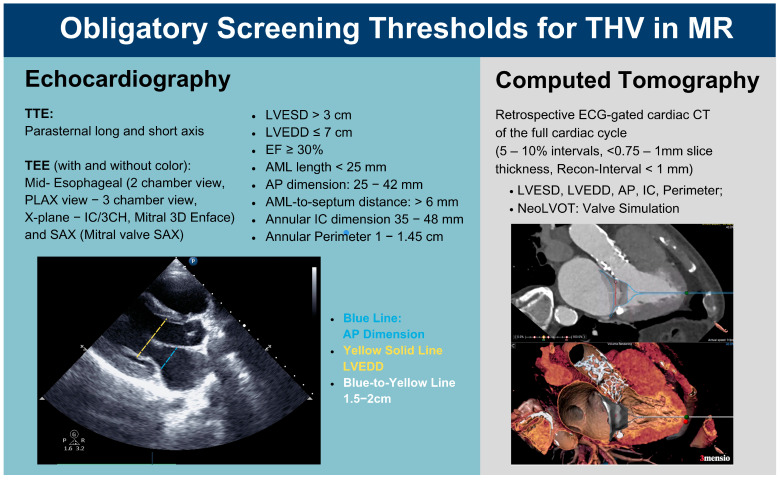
Obligatory screening thresholds for THV on MR. AML, Anterior Mitral Leaflet; AP, Anterior–Posterior; CT, Computed Tomography; EF, Ejection Fraction; IC, Intercommissural; IC/3CH, Intercommissural 3 Chamber View; LVEDD, Left Ventricular End-Diastolic Diameter; LVESD, Left Ventricular End-Systolic Dimension; MR, Mitral Regurgitation; NeoLVOT, Neo Left Ventricular Outflow Tract; PLAX, Parasternal Long-Axis; SAX, Short-Axis; TTE, Transthoracic Echocardiography; TEE, Transesophageal Echocardiography; THV, Transcatheter Heart Valve (Abbott Prescreening Guides based on [12,13,14], created unsing Canva).
